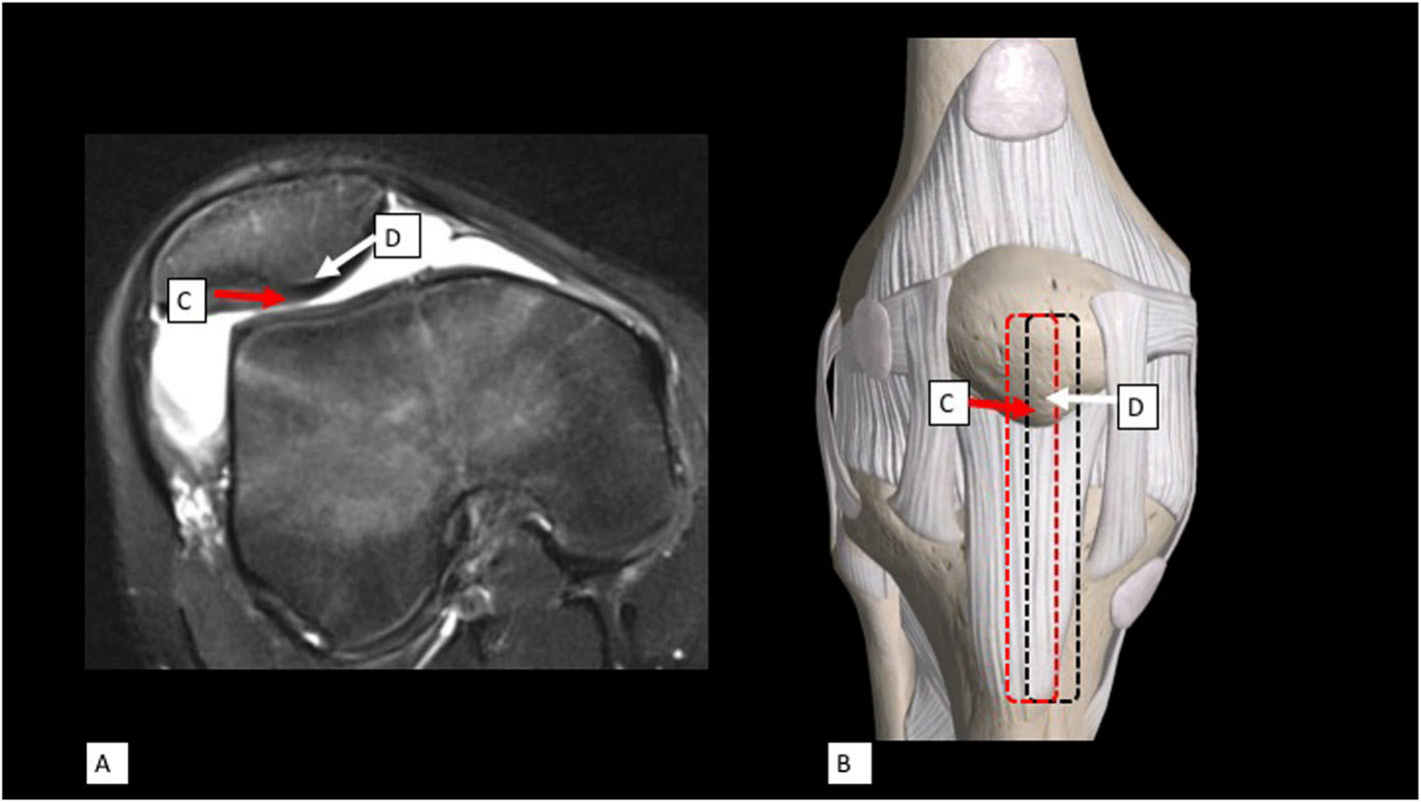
Method of pre-operative measurement of the harvesting area for bone-patella-tendon autograft. a Axial MRI of the right knee of a young male with anterior cruciate ligament (ACL) injury. Measurement of the distance from the mid-patella (c) to the central-ridge (d). b During the surgical procedure, the distance from the mid-patella to the central-ridge was measured (c, d). The new harvesting area (black dashed line) was shifted from the previous mid-patella area (red dashed line)
